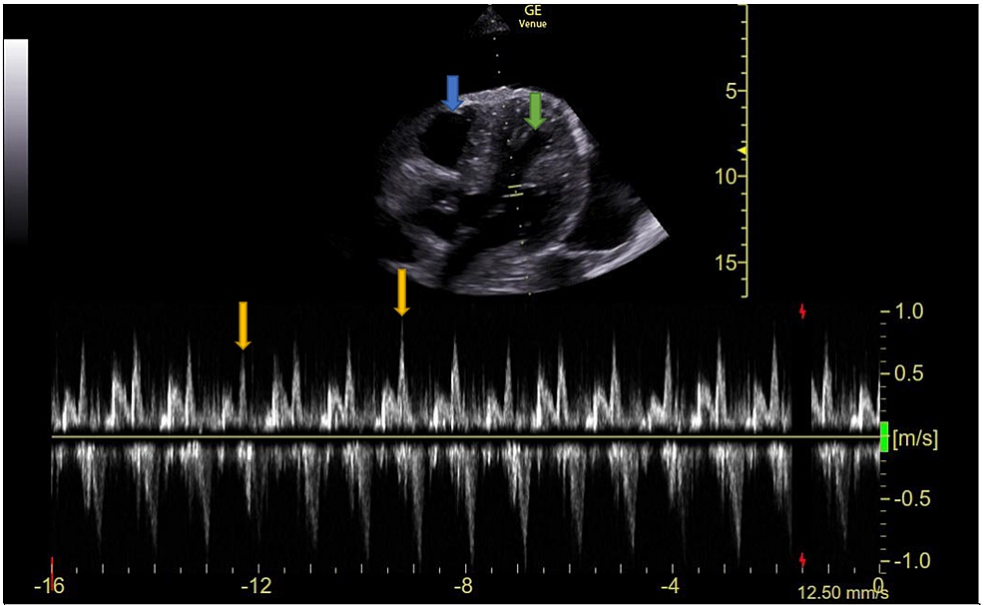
Echocardiography with pulse wave Doppler at the mitral valve showing ventricular interdependence, concerning for tamponade physiology Orange arrows show greater than 25% variability of passive filling of the left ventricle during the respiratory cycle. Blue arrow: right ventricle. Green arrow: left ventricle. Yellow brackets: mitral valve
